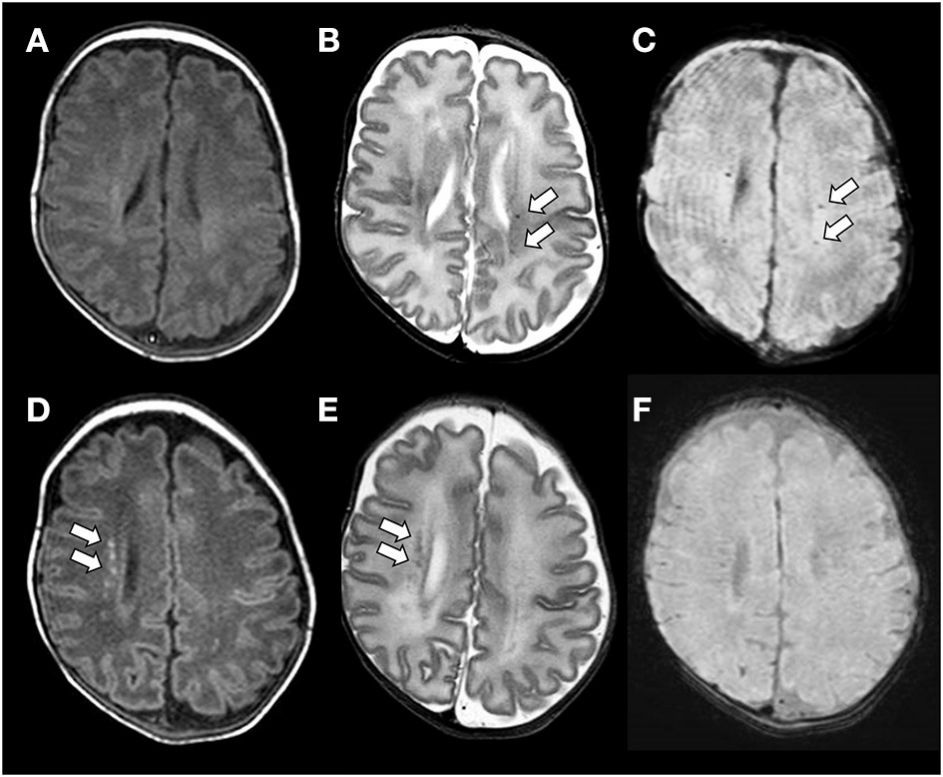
(A–C) Brain magnetic resonance imaging (MRI) performed at the term-equivalent age of an ex-preterm infant born at 25 weeks of gestation showing SWI+ PWMLs. SWI+ lesions are barely detectable on the axial T1-weighed image (A), whereas they are clearly visible as hypointense spots on axial T2-weighted (B) and SWI (C) sequences. (D–F) Brain MRI performed at term-equivalent age of an ex-preterm infant born at 29 weeks of gestation showing SWI– PWMLs. SWI- lesions are more clearly defined on the axial T1-weighted (D) than on the axial T2-weighted image (E) and are not visible on SWI (D).
